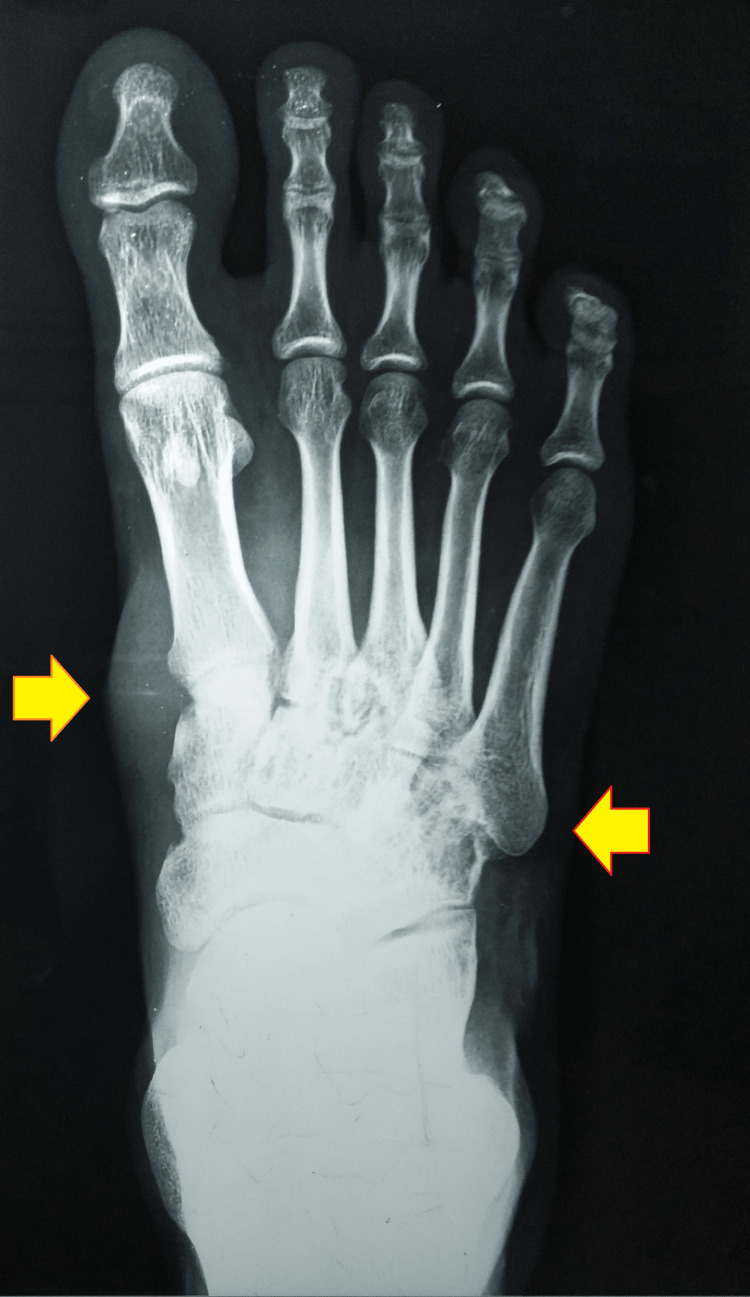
Anteroposterior radiograph of the right foot showing soft tissue swelling and bony involvement
